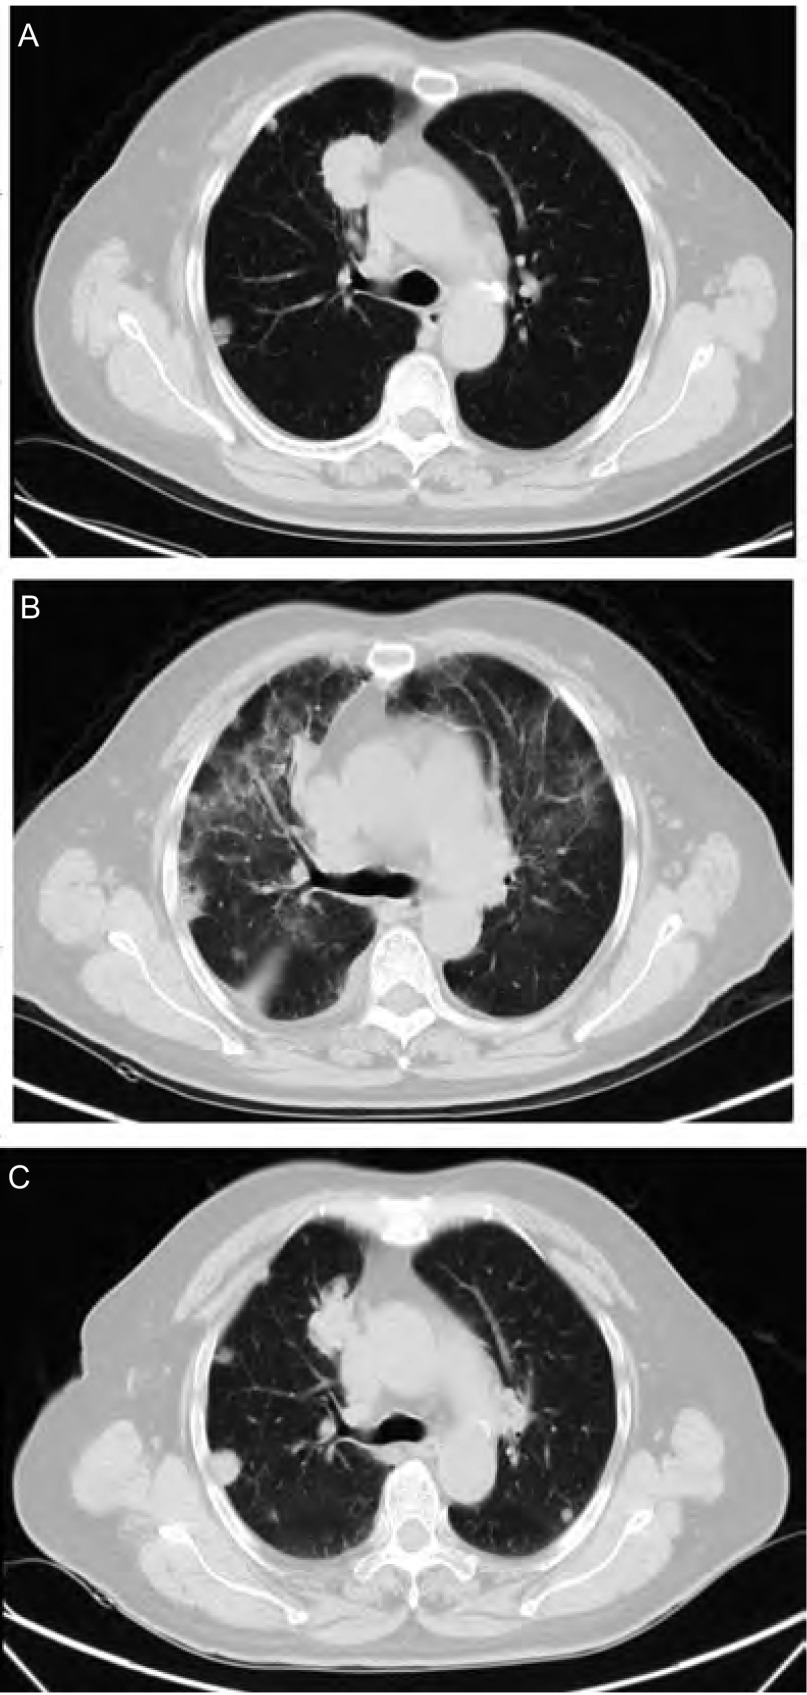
病例2厄洛替尼治疗前后及糖皮质激素治疗后胸部CT变化 Chest CT scans before and after erlotinib treatment and after corticosteroid treatment. A: Chest CT scan before erlotinib treatment; B: Chest CT scan after 70 days of erlotinib treatment; C: Chest CT scan after 10 days of corticosteroid treatment.
